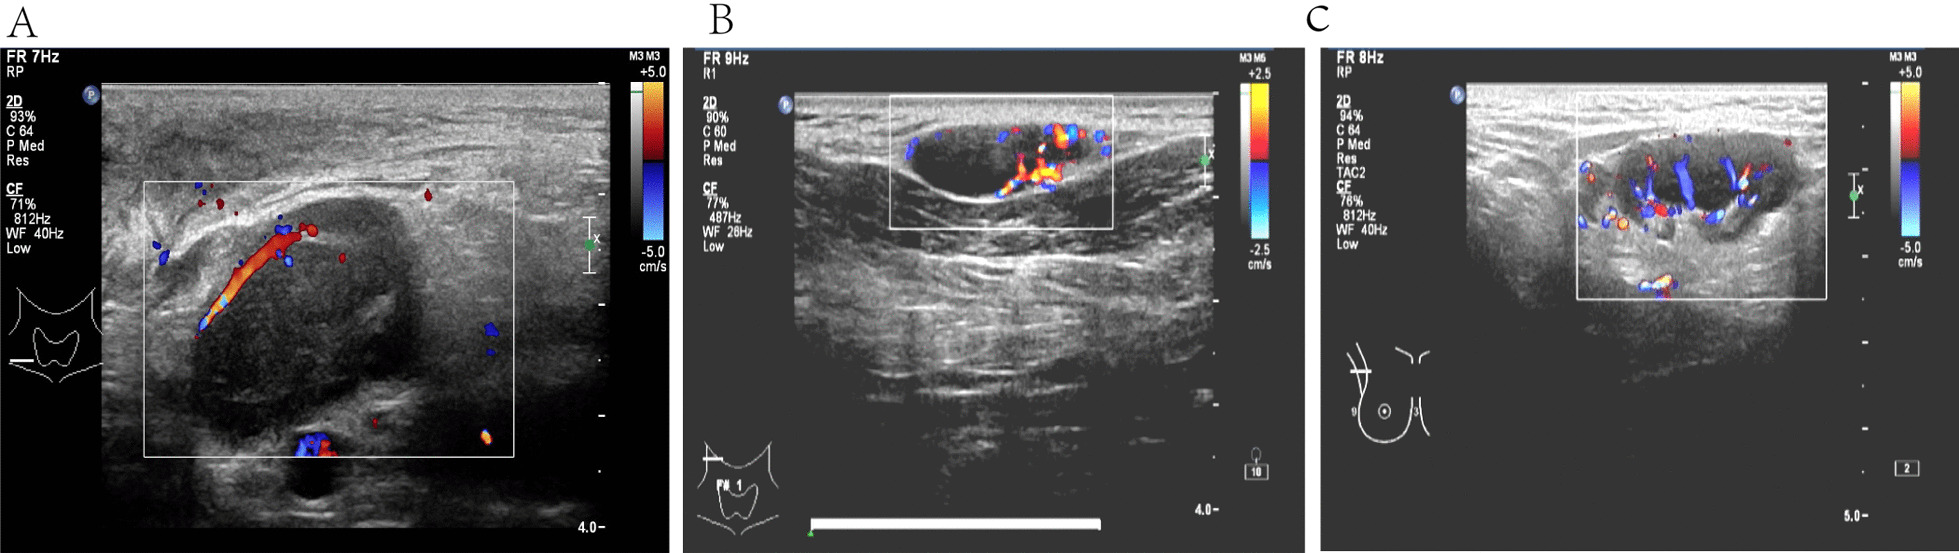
A Color Doppler flow image of tuberculosis in the right cervical lymph nodes, strip-shaped blood-flow signals could be seen at the margins. B Color Doppler flow image of tuberculosis in the right cervical lymph nodes, mixed-type blood-flow signals. C Color Doppler flow image of a patient with lymphadenitis in the right axillary lymph node, central-type blood-flow signals
